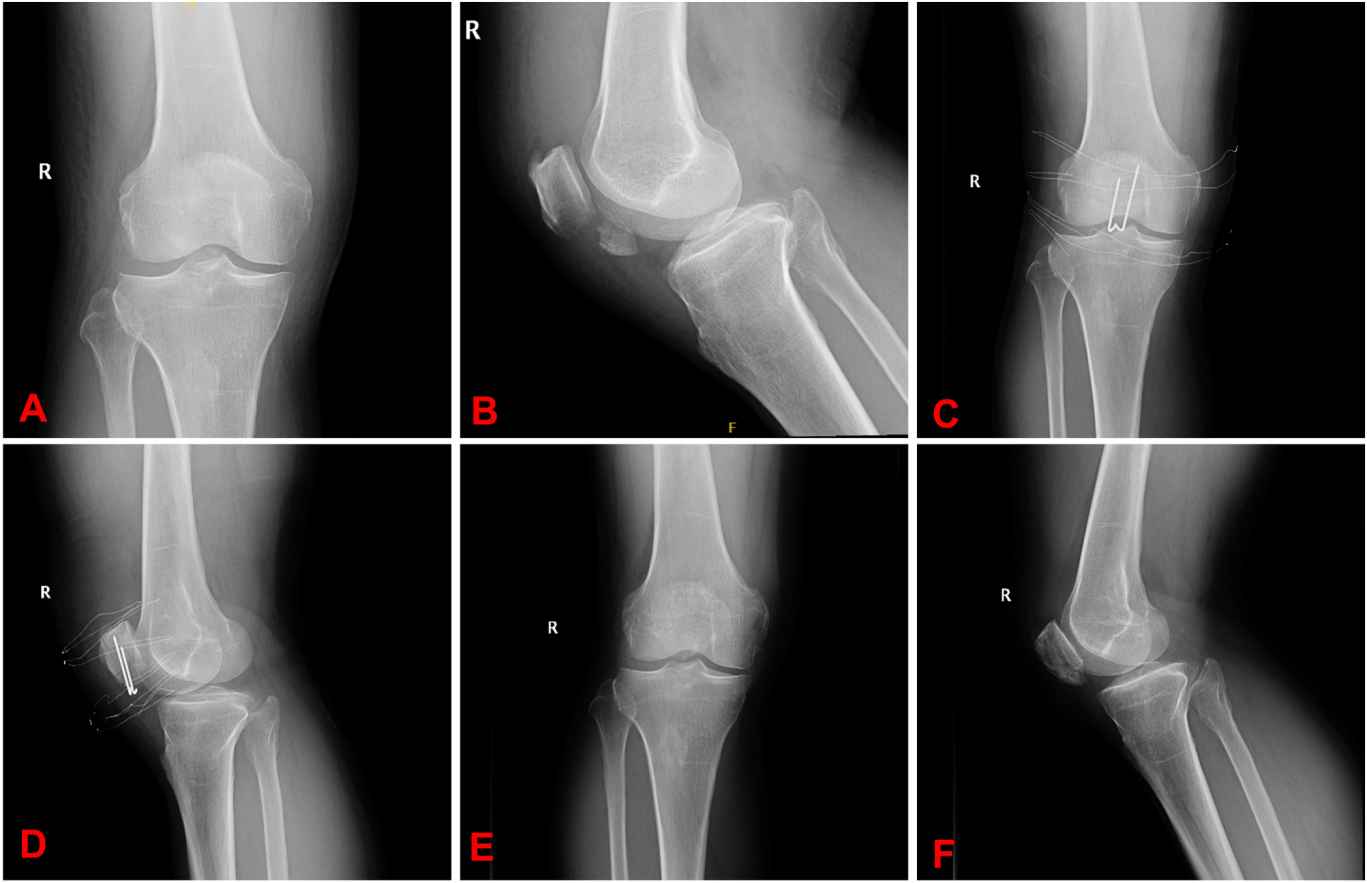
(A,B) Radiographs of distal patellar fracture. (C,D) In this distal patella fracture, we used the 5-Ethibond and three K-wires. (E,F) Radiograph after implant removal.
